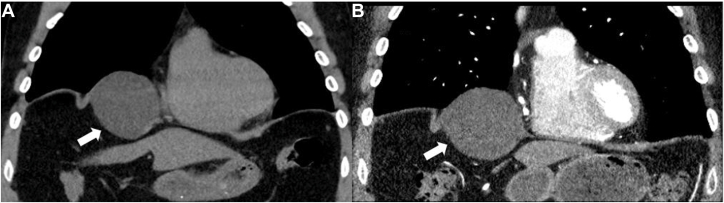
Computed Tomography Coronal View Computed tomography coronal view of the diaphragmatic mesothelial cyst (arrow) in (A) 2018 and (B) 2025. The lesion has grown slightly overtime. There is cranial displacement of the diaphgram along the lateral edge (rightward). This favors extrapericardial and diaphragmatic rather than pleural source of origin.
